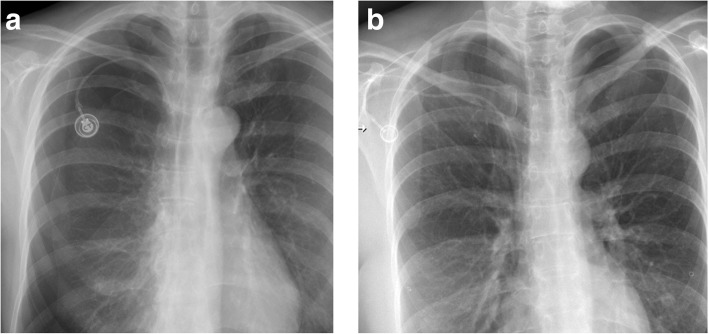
Pneumothorax. a Three days after correct placement of port system, the patient complained of dyspnea. Chest radiograph shows a large right pneumothorax. b Regular placement of a port system on the right side. There is a contralateral pneumothorax from unsuccessful attempts on the left side, which were not mentioned in the referral
